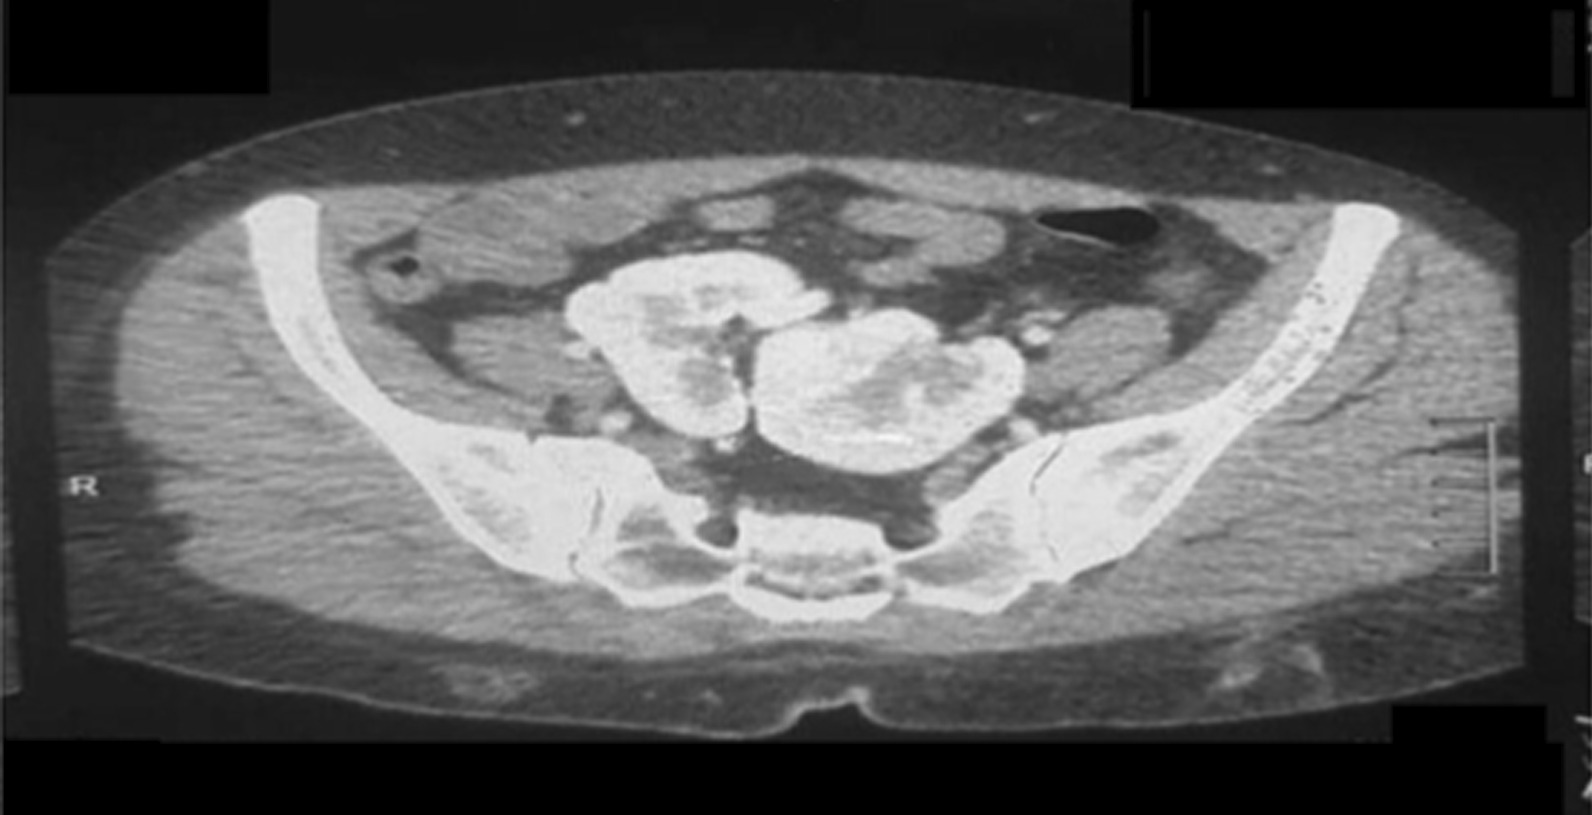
Computed tomography urography. The right kidney is ectopically placed in the pelvis, measures 9.6 cm bipolar length, and is medially and inferiorly faced. The left kidney is also ectopically placed in the pelvis, measures 9.3 cm in bipolar length, and is mal-rotated as the pelvis faces upward and laterally, both kidneys are partially fused at their upper poles
